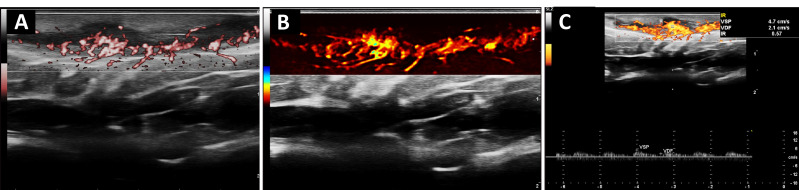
Doppler imaging was performed using the same acquisition parameters as those described in Figure 2 (high-frequency linear probe at 12.5 MHz; PRF of 480 Hz optimized for low-velocity flow detection). (A) Power Doppler imaging demonstrates a hypoechoic inflammatory sinus tract (subcutaneous tunnel) with prominent intralesional and perilesional vascular signals, consistent with active inflammatory involvement. (B) Advanced Doppler modality (Microvascular/Enhanced Doppler highlights dense low-velocity vascular networks within the tract wall, reflecting inflammatory angiogenesis and microvascular remodeling. (C) Spectral Doppler interrogation of an intralesional vessel shows a low-resistance arterial waveform (VSP 4.7 cm/s; VDF 2.1 cm/s; RI 0.57), supporting active inflammatory hyperemia. These multimodal Doppler findings provide structural and hemodynamic characterization of sinus tract activity, consistent with Doppler-based inflammatory biomarkers described in HS [4,5,6].
